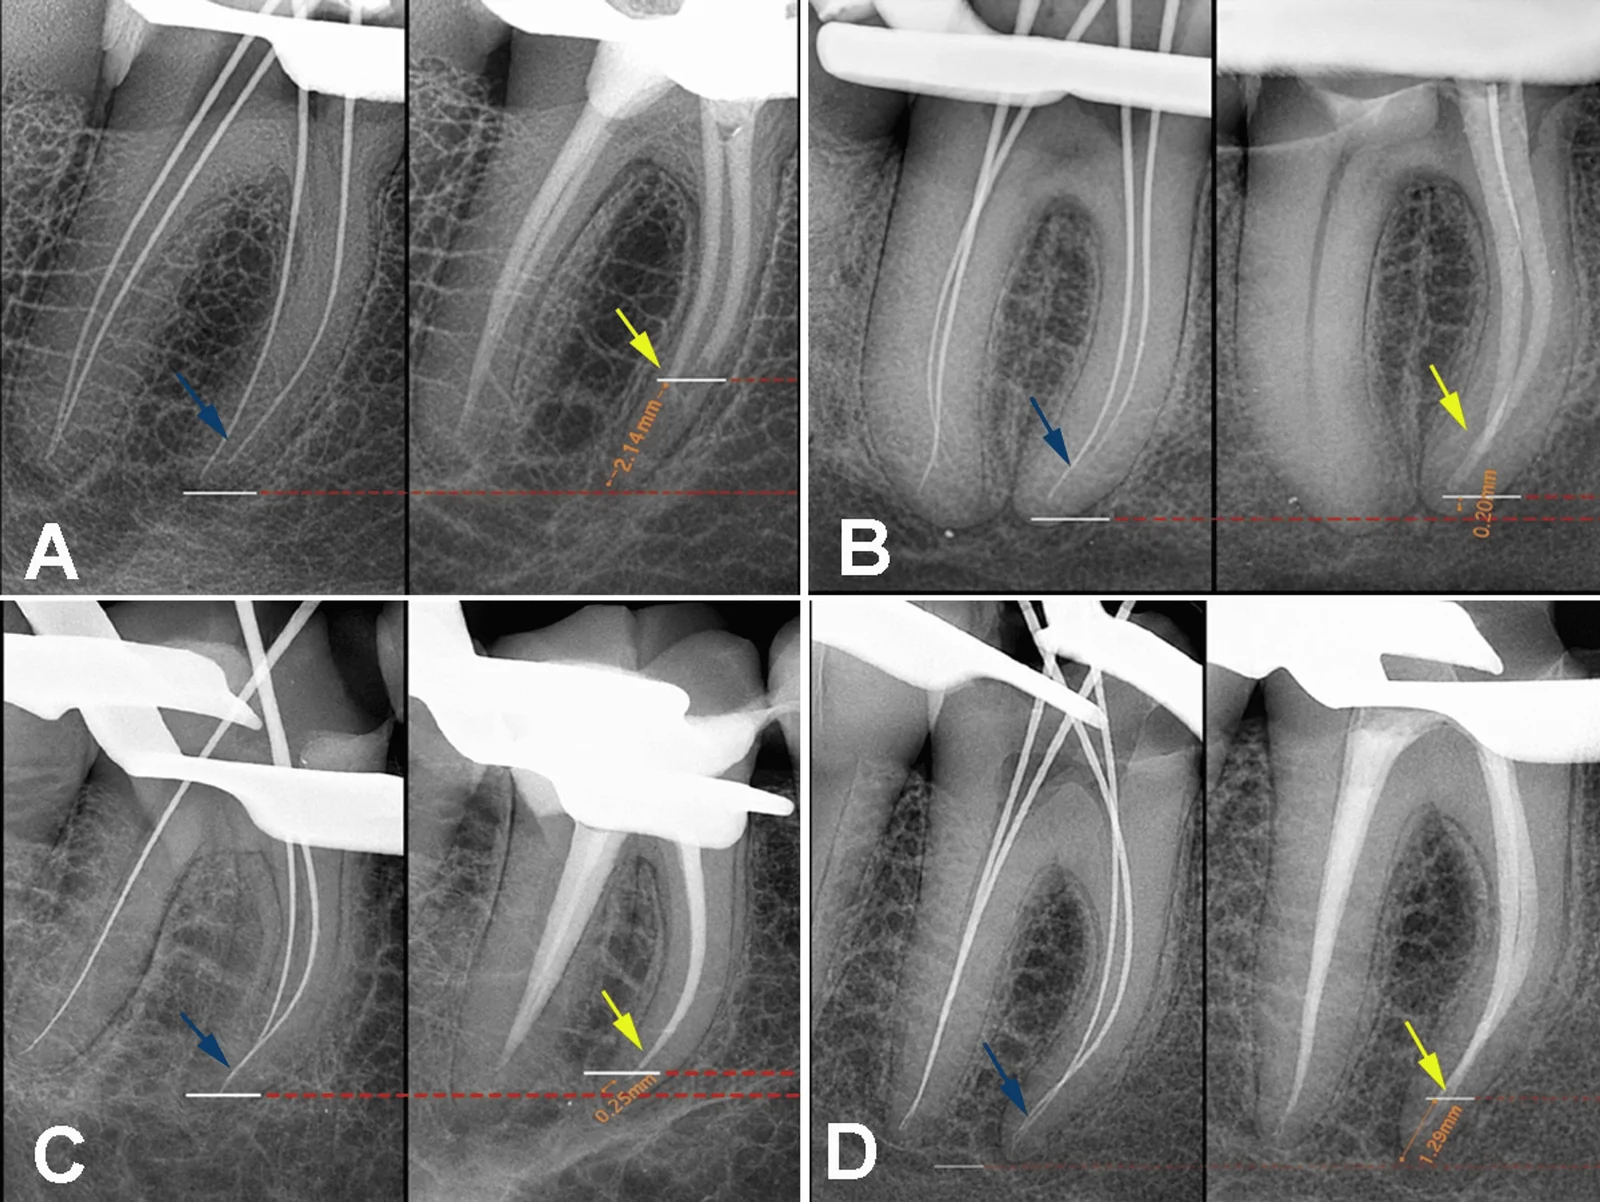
Intraoral periapical radiograph showed canal preparation, followed by irrigation with different techniques and infiltration with dye (A) Diode laser-assisted irrigation, (B) PATS air-sonic, (C) Sonic, (D) Ultrasonic. Blue arrow: Endodontic file in mandibular first molar canal (mesial canals). Yellow arrow: Dye infiltrate in mandibular first molar canal (mesial canals). PATS: Pro-Agitator Tip System
